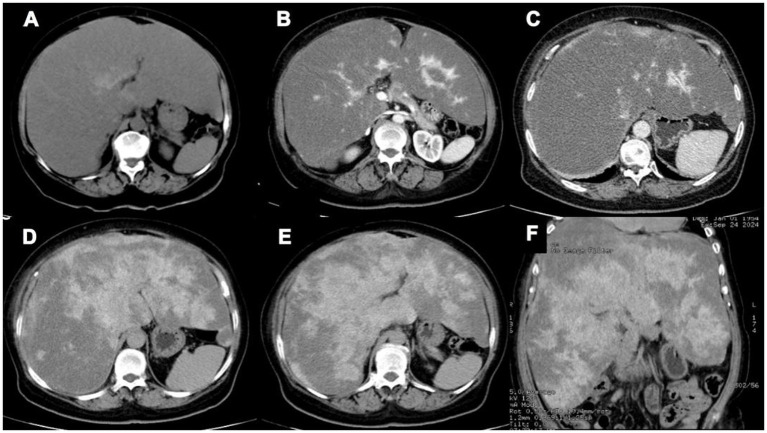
Plain and contrast-enhanced abdominal CT. Reduced hepatic parenchymal density with wavy hepatic margin (A); heterogeneous enhancement of portions of the hepatic parenchyma (B); slender intrahepatic segment of the inferior vena cava, attenuated left and middle hepatic veins, and indistinct right hepatic vein (C); heterogeneous hepatic parenchymal density on delayed-phase imaging with a “geographic map–like” heterogeneous enhancement pattern (D,E); markedly enlarged liver extending to the iliac fossa (F).
